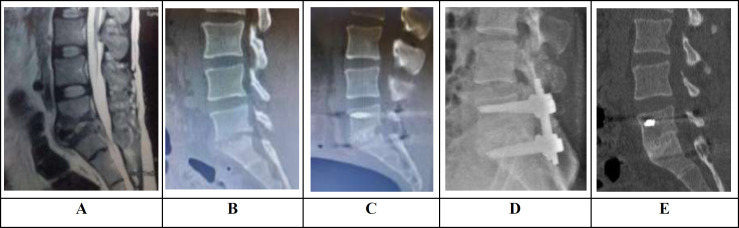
Sagittal T2W lumbosacral MRI (A) and sagittal lumbosacral CT (B) showed preoperative findings of spondylodiscitis at L5-S1. The operation was carried out with posterior instrumentation and allograft autograft combination bone chips interbody fusion at L5-S1 level (C). At 25 months after surgery, lateral X-ray (D), and sagittal lumbosacral CT (E) showed rigid fixation and fusion without any evidence of pseudoarthrosis
